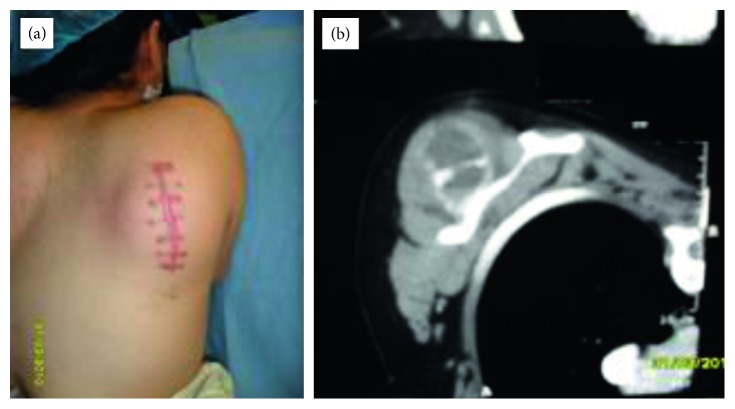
(a) Posterior Scapular mass with broad scar after resection attempt. (b) Axial CT showing soft tissue mass extending posterior to the scapula.
